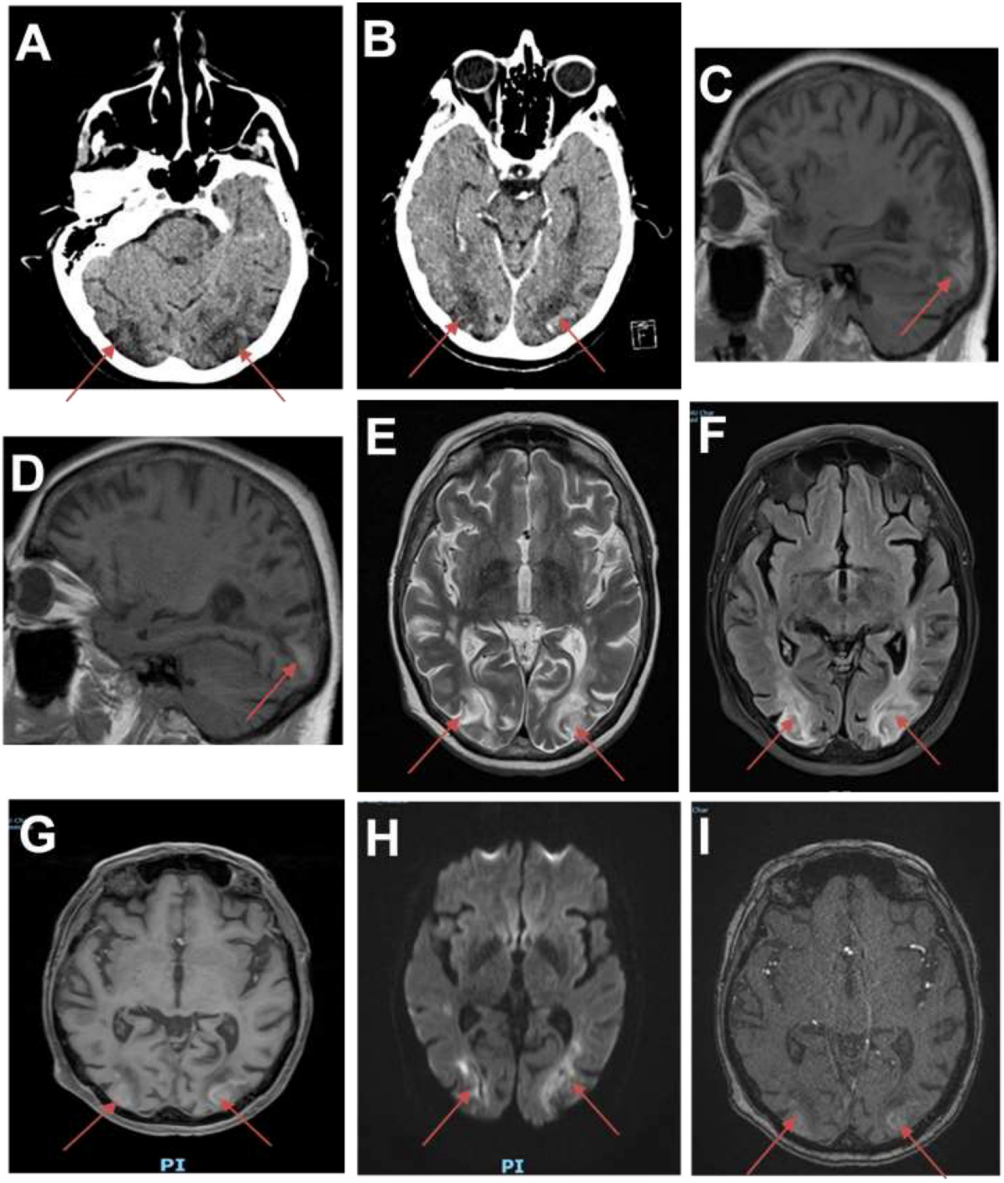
First brain imaging. (A) Brain CT showing ischemic-like lesions in the occipital lobes (red arrows). (B) CT scan showing hypodense lesions with breakdown of the blood–brain barrier (BBB) in the occipital lobes. (C,D) Sagittal T1 MRI showing hyperintensities in both occipital lobes. (E) Brain MRI (T2-weighted images) showing hyperintense lesions in the white matter of the occipital lobes. (F) Fluid attenuation inversion recovery (FLAIR) images showing ischemic-like lesions in the occipital lobes. (G) MRI angiographic sequences showing breakdown of BBB in the occipital lobes. (H) Diffusion sequence showing restriction in the occipital lobes. (I) Magnetic resonance angiography time-of-flight (MRA TOF) sequences showing breakdown of BBB in the occipital lobes.
